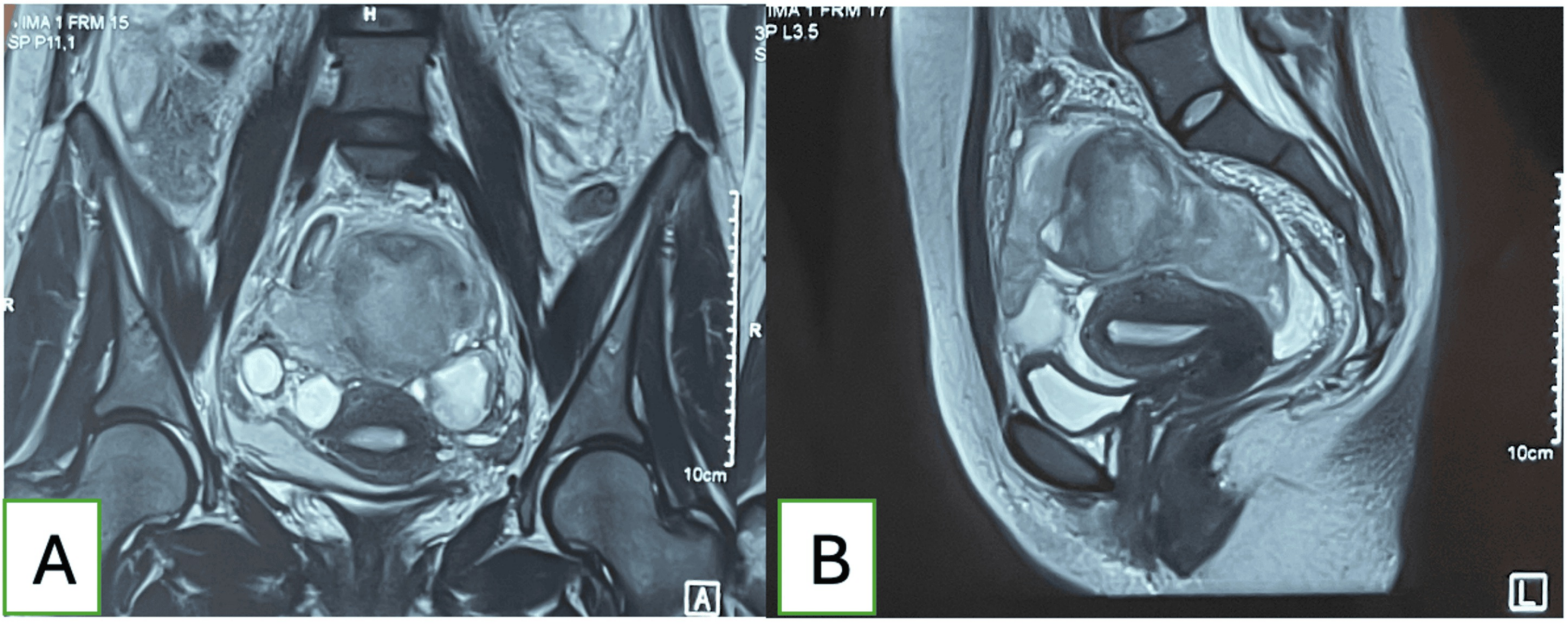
MRI images showing moderate hematic pelvic effusion with a left hemorrhagic ovarian cyst (A) Axial view showing a left hemorrhagic cyst and right hydrosalpinx. (B) Sagittal view showing moderate hematic pelvic effusion and a suprauterine hematoma.
